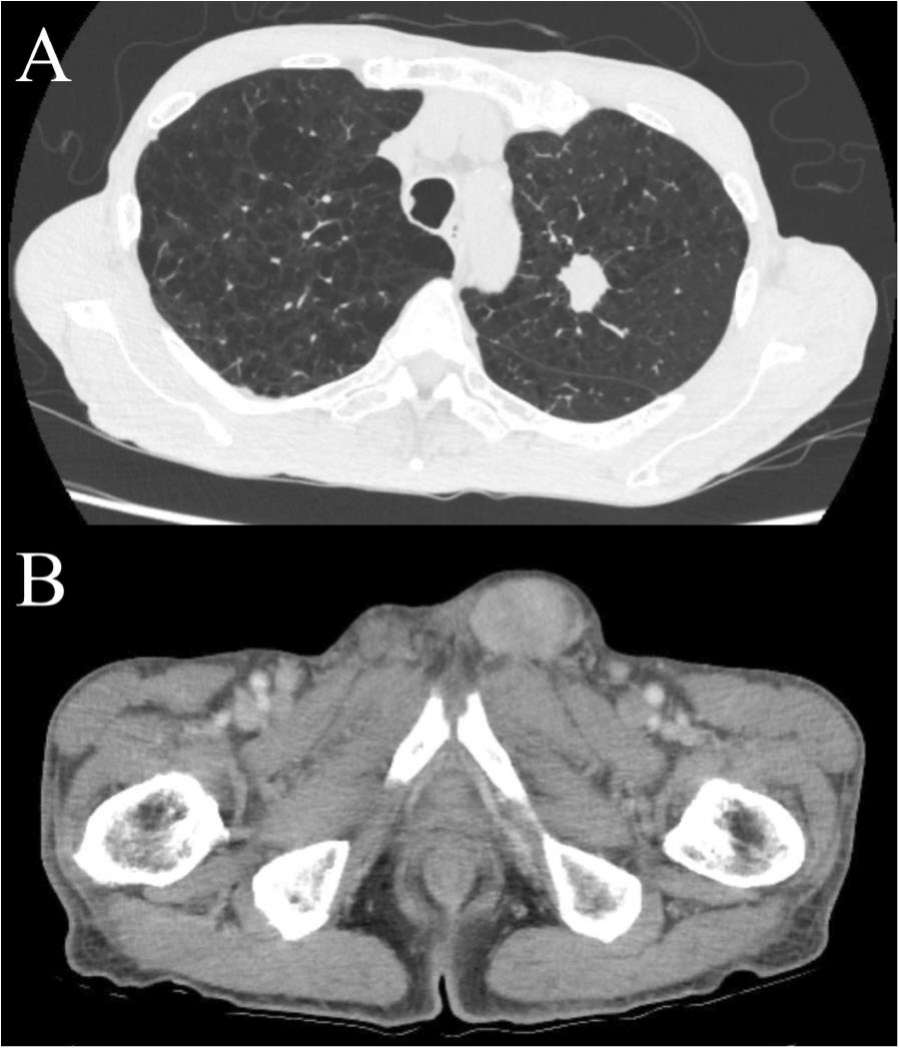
Computed tomography findings of the pulmonary nodule and inguinal solid mass. A computed tomography scan reveals a pure-solid pulmonary nodule (23 × 20 × 25 mm) in the left upper lobe (A) and solid mass (50 × 30 × 55 mm) in the left inguinal area (B)
